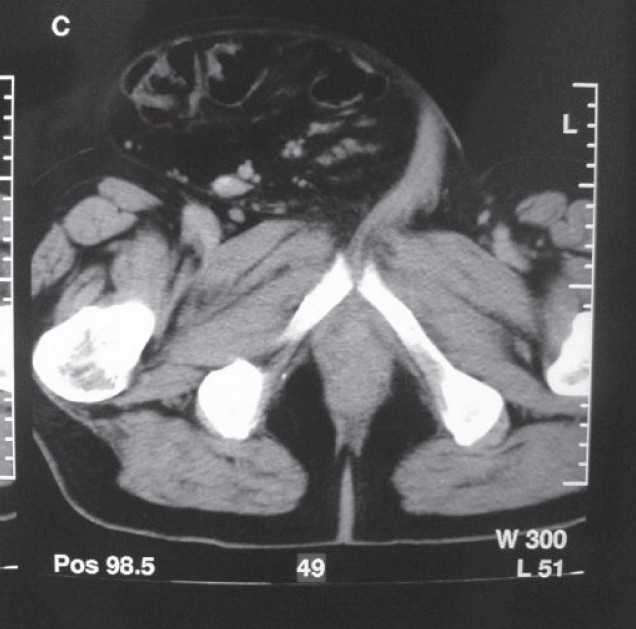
Preoperative computed tomography of the second patient.
